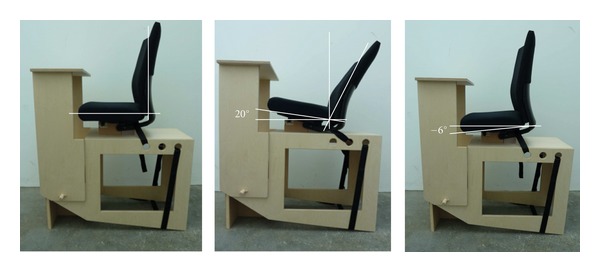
MRI-compatible chair in the three positions: upright (left), reclined (middle), and forward inclined (right).
